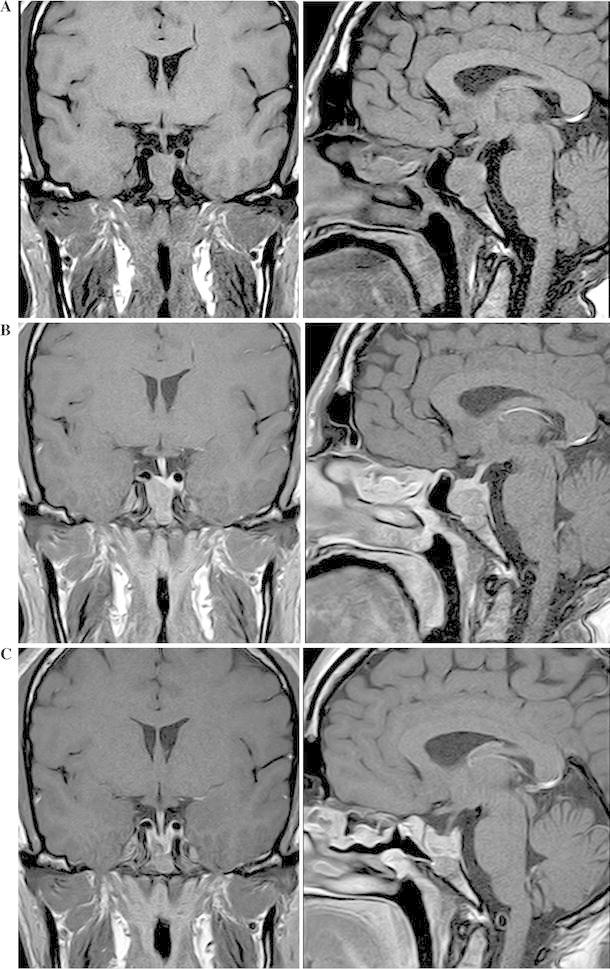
Case study: post-contrast, T1-weighted MRI scans of pituitary tumor at diagnosis (a), before surgery (b) and three months after surgery (c) (coronal* left*, sagittal* right*)Diagnosis
